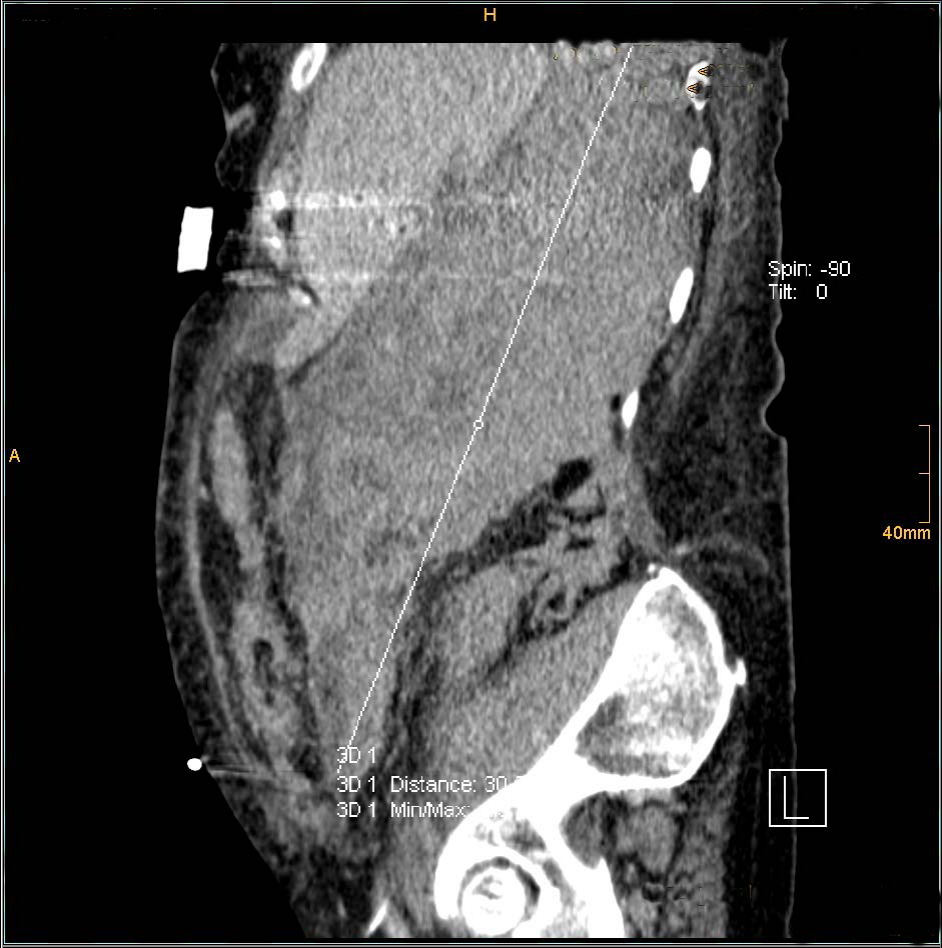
3^rd ^case. CT - sagittal plan demonstrating a 30 cm hematoma.
